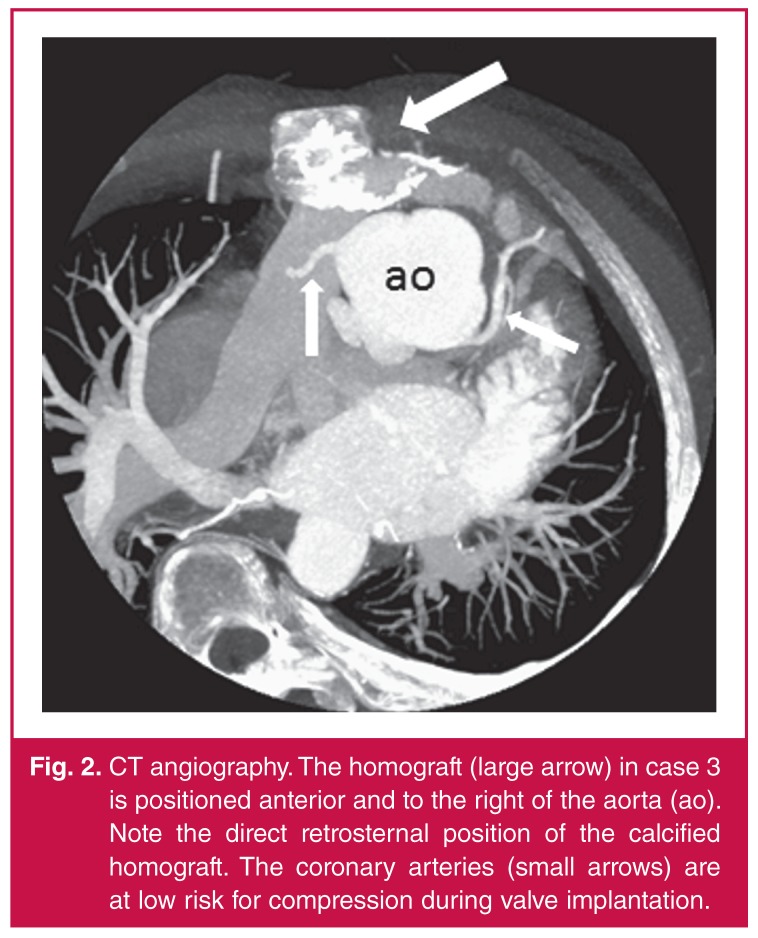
CT angiography. The homograft (large arrow) in case 3 is positioned anterior and to the right of the aorta (ao). Note the direct retrosternal position of the calcified homograft. The coronary arteries (small arrows) are at low risk for compression during valve implantation.
